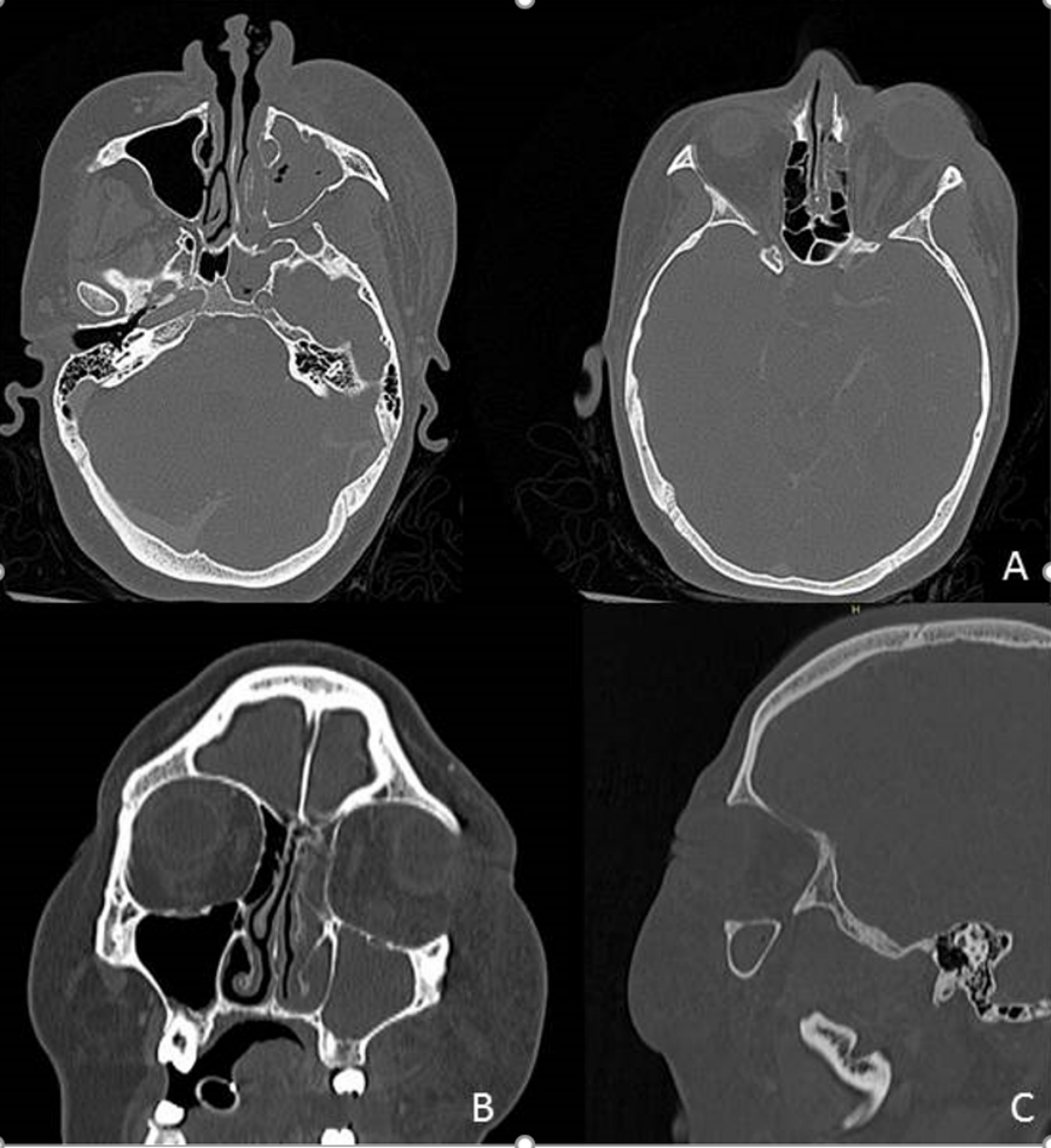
Contrast enhanced CT showing occupancy of the left maxillary, ethmoid, and sphenoid sinuses, without apparent intracranial involvement. Striation of the extraconal fat is observed in the lower left region, as well as periorbital and ipsilateral facial region with associated proptosis. Axial (A), coronal (B) and sagittal (C).
